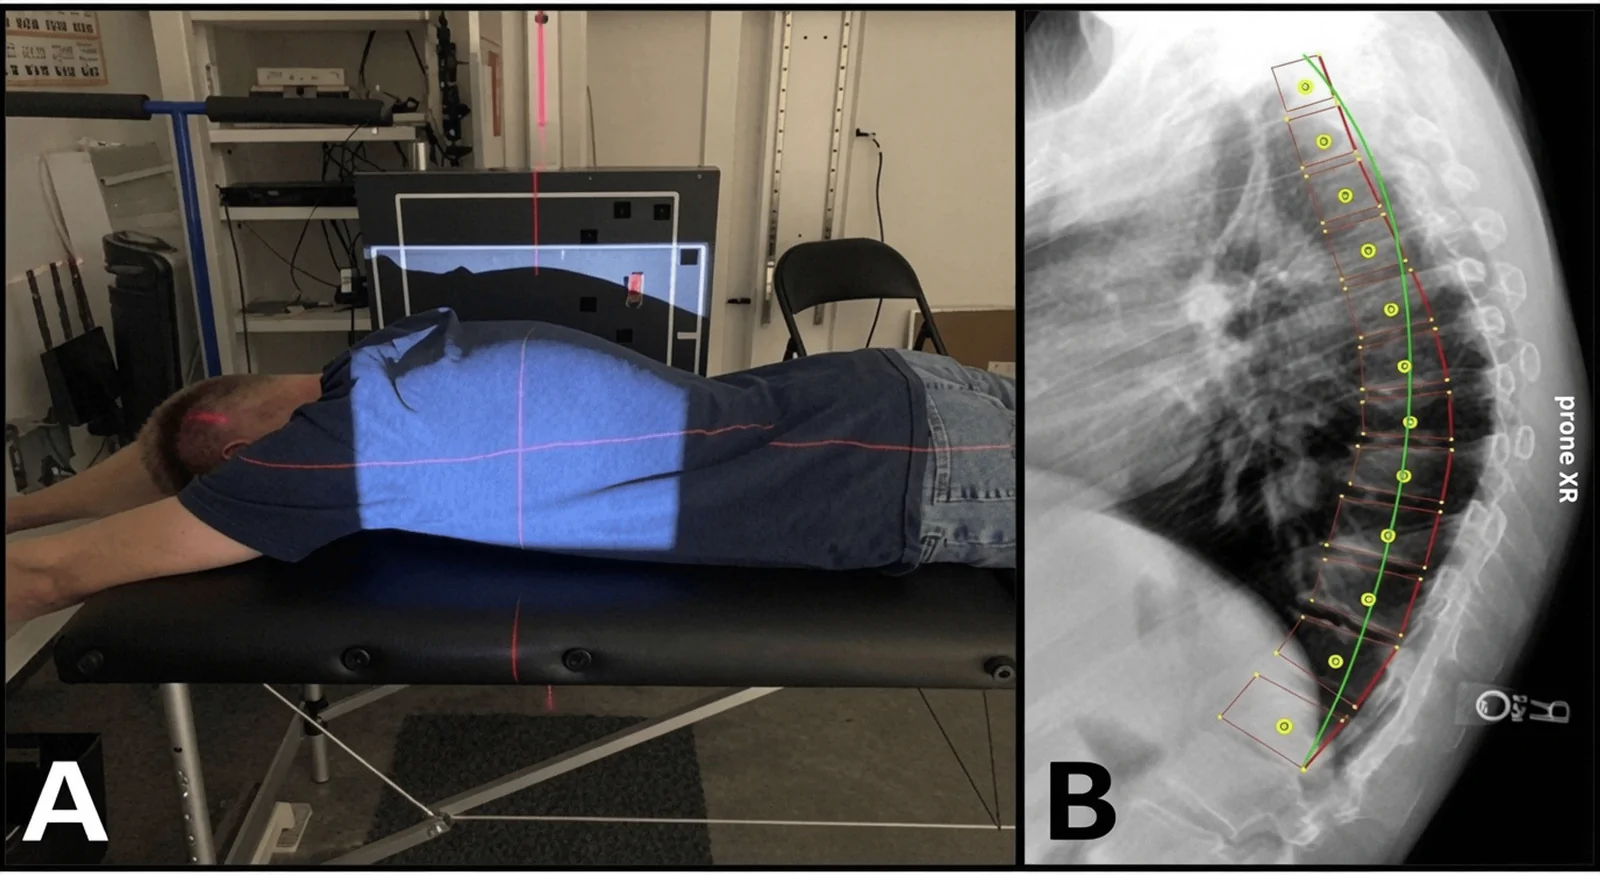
Thoracic spine flexibility prone radiograph positioning and results. A-B) Prone postural correction potential position showing lateral thoracic radiograph position and image results. The patient was placed in the prone position to ascertain the potential correction and overall flexibility of the thoracic spine in the position that reduced the spine deformity. The light represents the frame of the radiograph and the patient was attempting to flatten the thoracic spine as his muscles would allow. The results are then applied to the therapeutic treatment regimen of MI® exercises, traction, and postural SMT.
